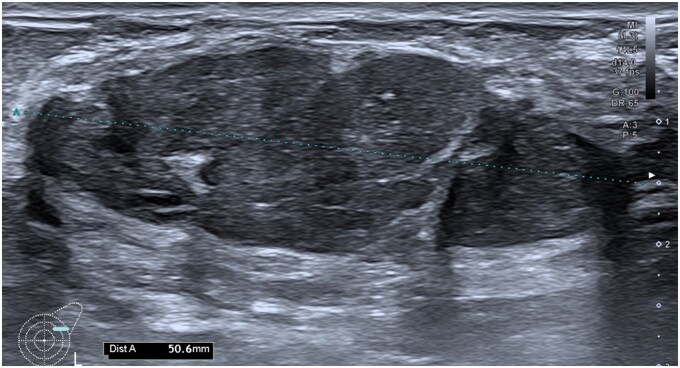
Shows an ultrasound image of a downgraded lesion (BPT to B2 FA). Neither size nor ultrasound score was predictive of downgrade.
